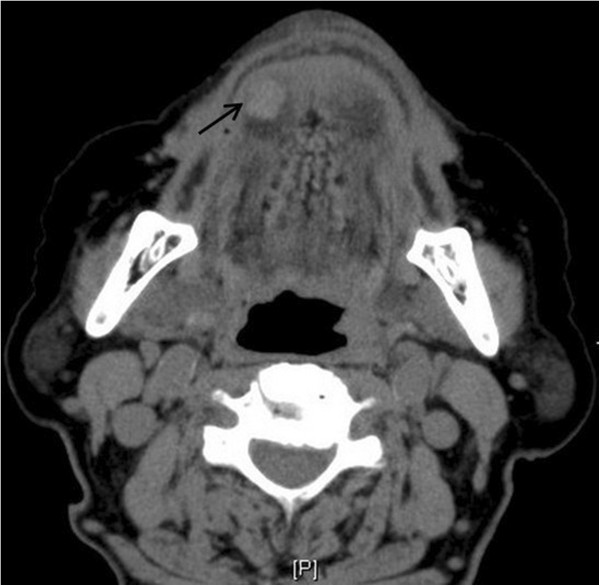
A spiral CT shows a round, well-circumscribed mass (arrow) in the right tip of tongue with homogeneous hypodensity compared with that of masseter.
